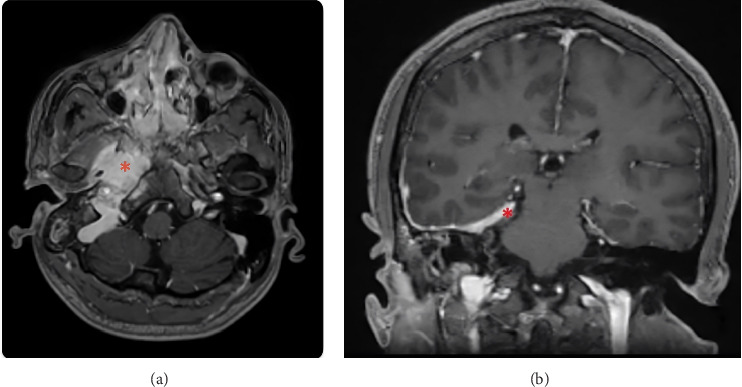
(a): Axial view of a T1 MRI with contrast showing enhancement along the right mastoid and petrous apex (highlighted with the asterisk (^∗^)). This was interpreted as concerning for mastoiditis and osteomyelitis. (b): Coronal view of a T1 MRI with contrast prior to treatment demonstrating dural thickening and enhancement along the skull base (asterisk (^∗^)), petrous apex, and mastoid.
